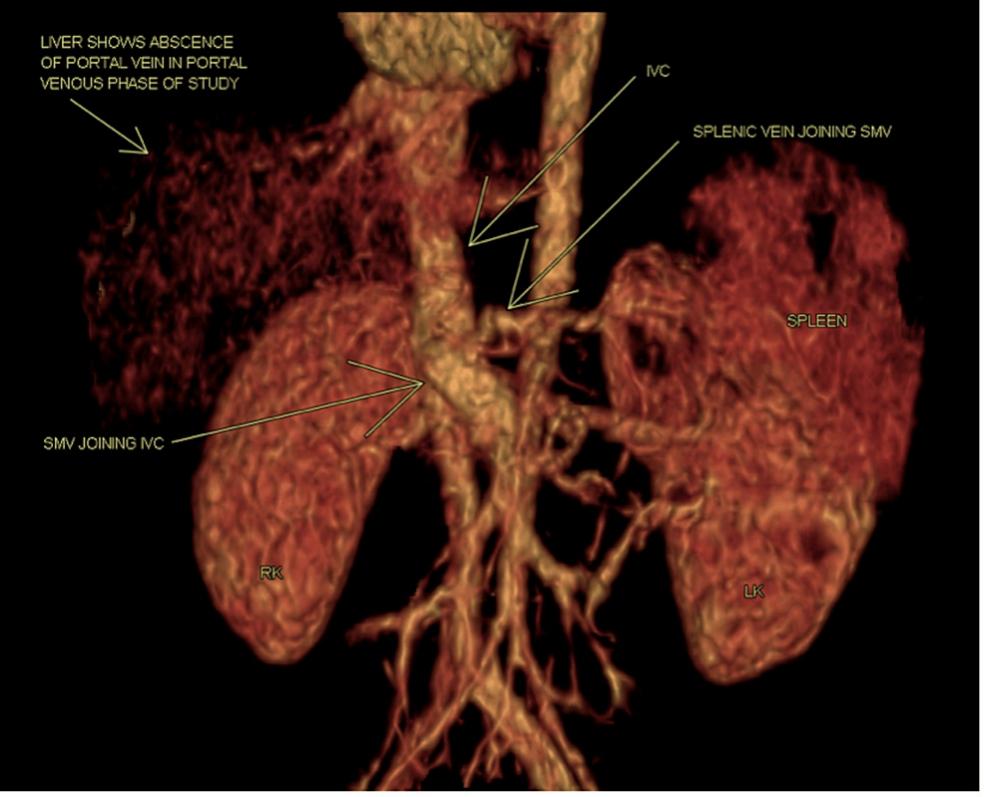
3D reconstruction CT of the portosystemic shunt (anterior view)- the superior mesenteric vein and the splenic vein are draining into the IVC with an absent portal vein. 3D: three-dimensional; CT: computed tomography; IVC: inferior vena cava; SMV: superior mesenteric vein
